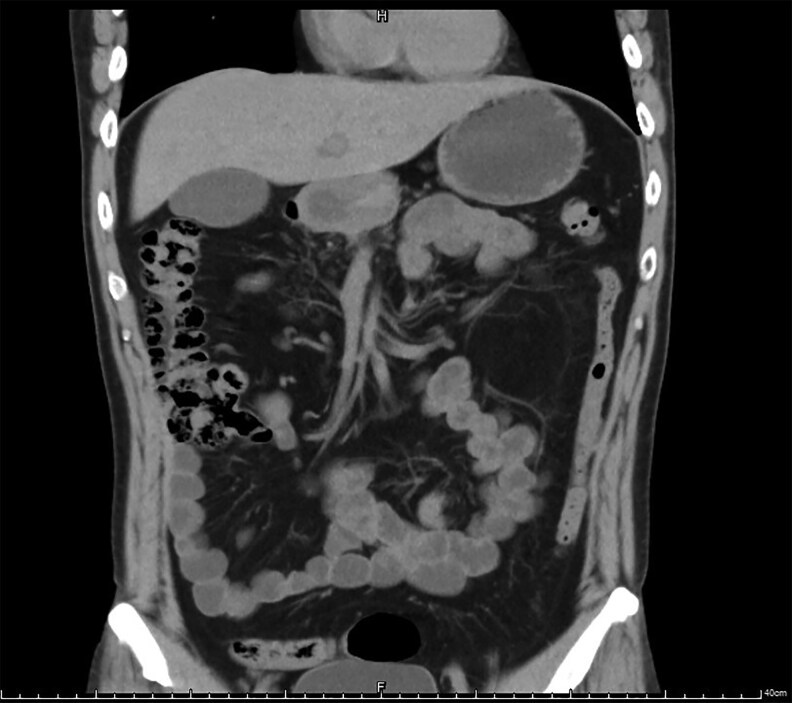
Abdominal computed tomography scans (coronal view), obtained 2 months after initial presentation and intervention, show complete resolution both of intramural air and free abdominal air.
